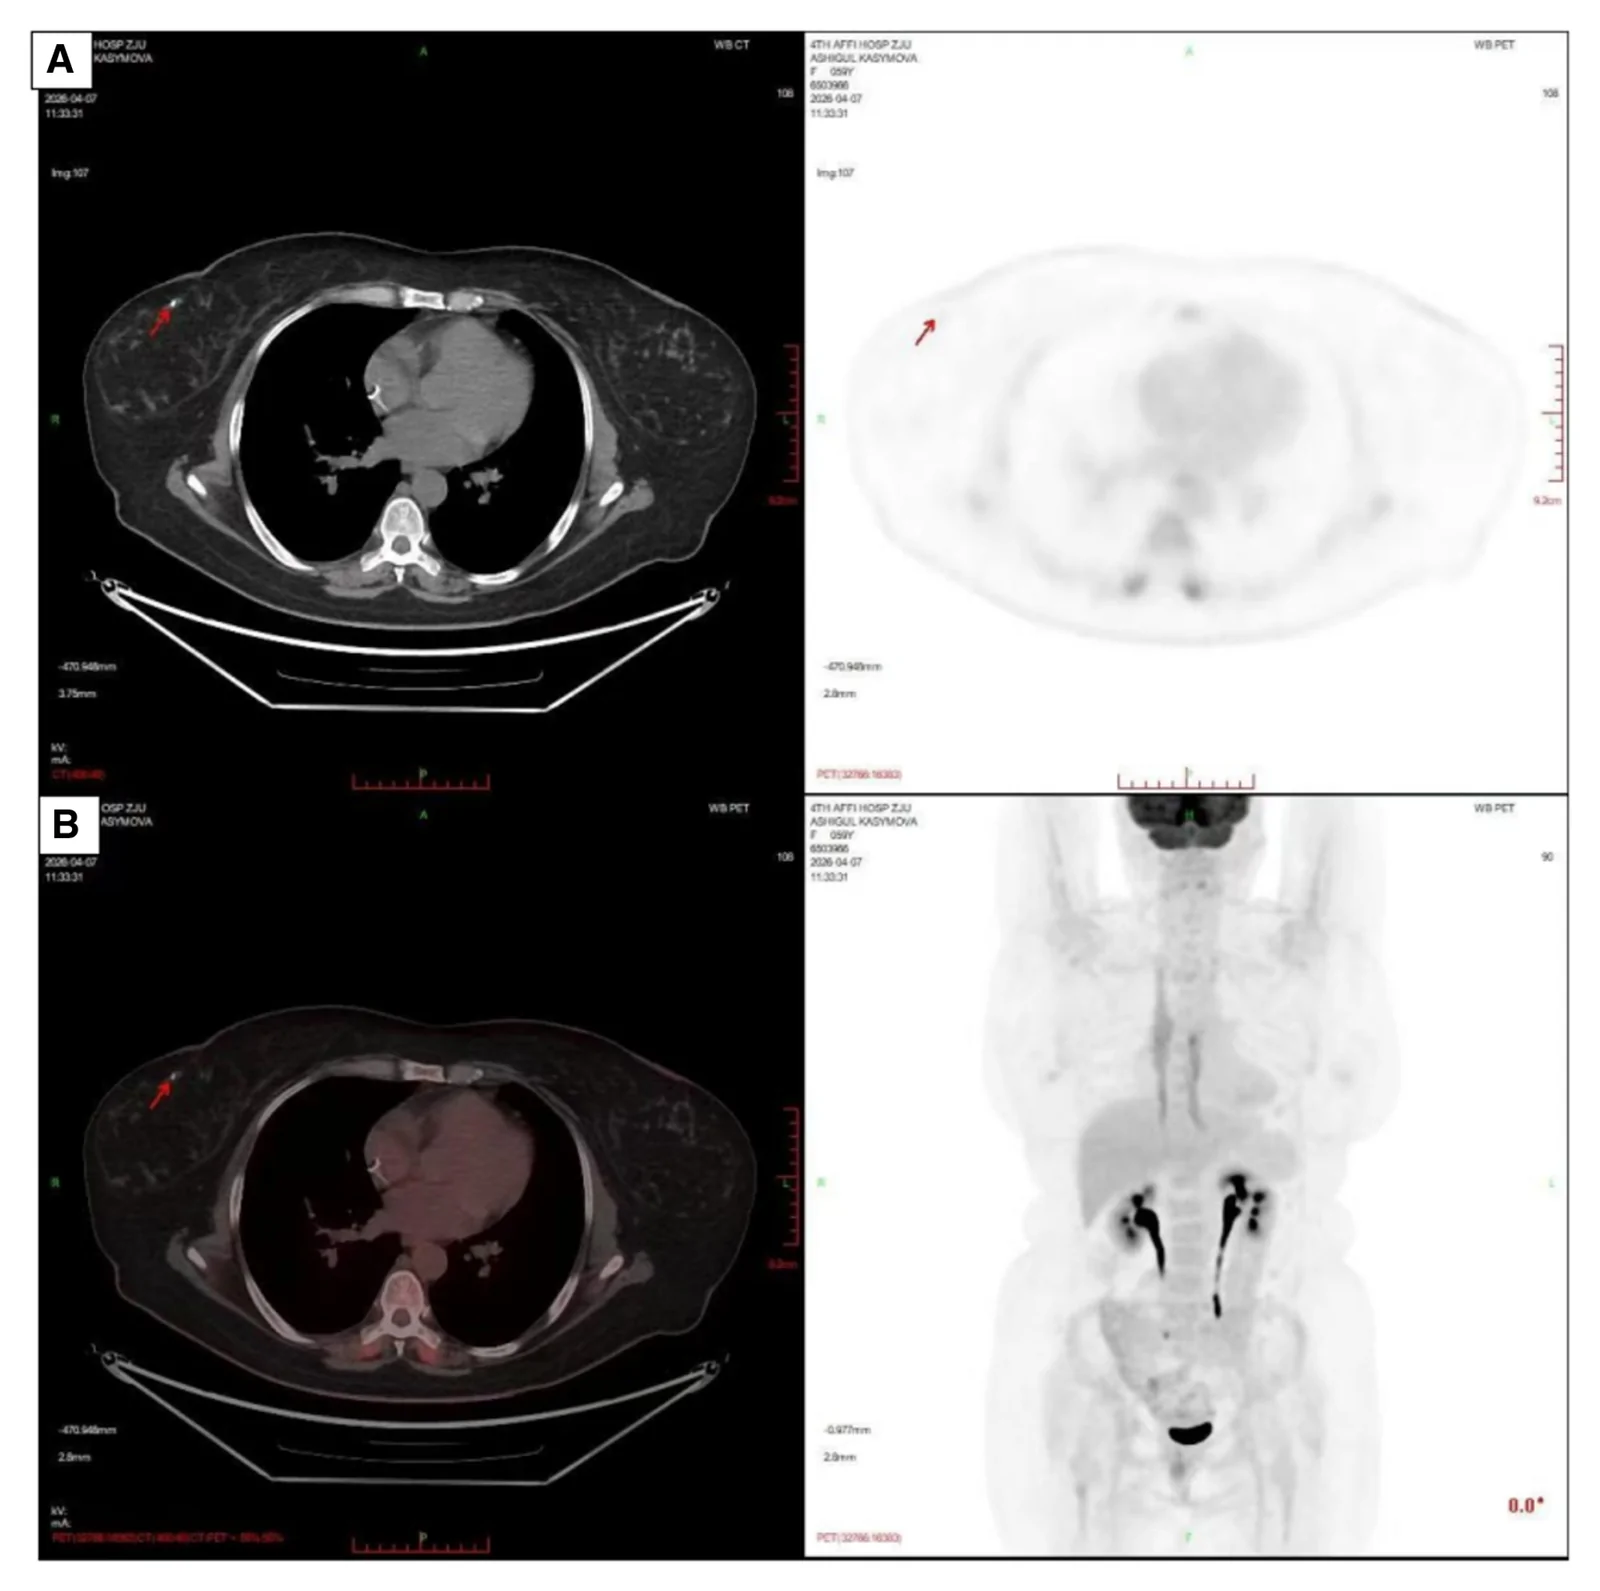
posttreatment follow-up PET/CT images of the patient. (A) PET/CT showed a punctate calcific density measuring approximately 0.4 × 0.4 × 0.3 cm in the upper-outer quadrant of the right breast (arrows). (B) No abnormal ^18F-FDG uptake (arrow).
